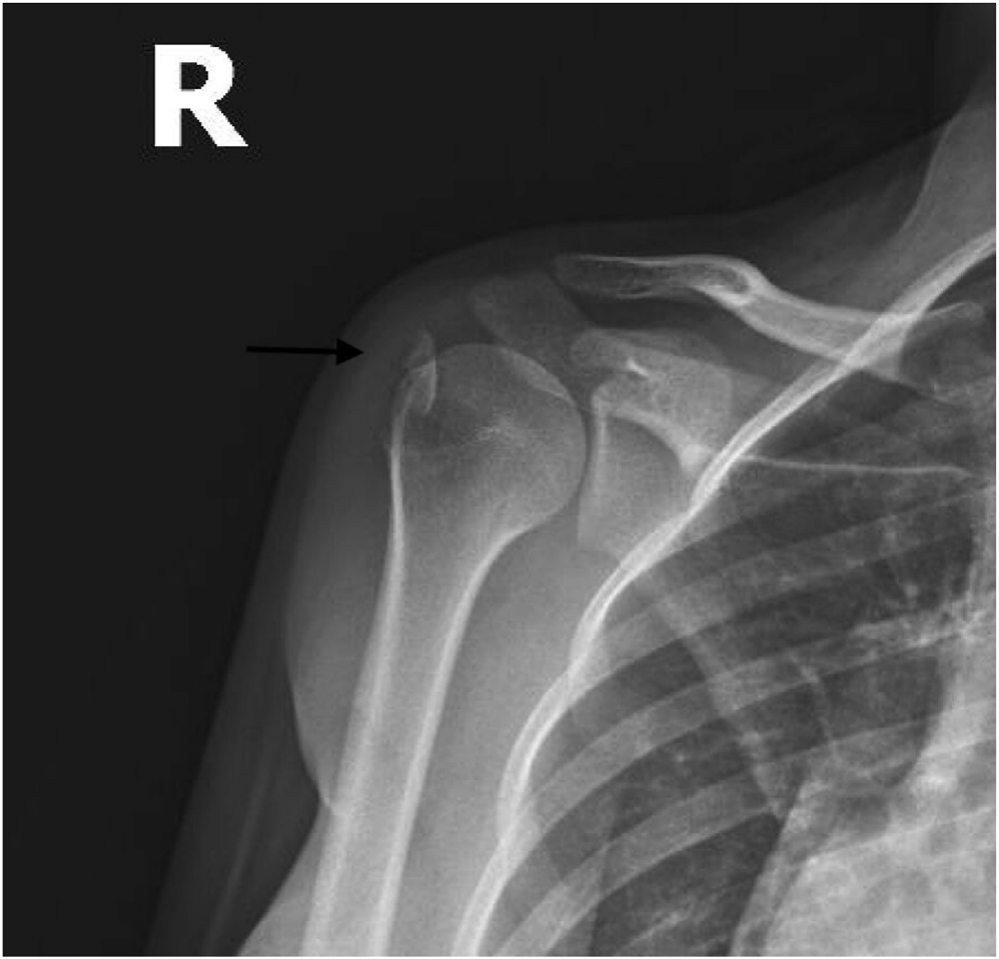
Anteroposterior radiograph showing a lesser tuberosity avulsion fracture (arrow). (R, right shoulder).
